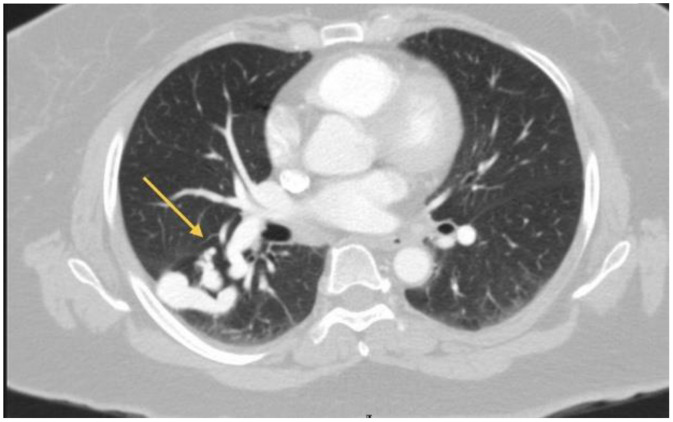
Chest CT scan with intravenous contrast, axial view, in the right middle lobe, adjacent to the medial segment, shows a serpiginous and aneurysmal vascular lesion (yellow arrow) with intense enhancement after administration of intravenous contrast, corresponding to a pulmonary arteriovenous malformation (arteriovenous fistula). A dilated arterial afferent vessel, a well-defined aneurysmal sac, and its corresponding draining vein into the pulmonary venous circulation are clearly visible in this phase.
